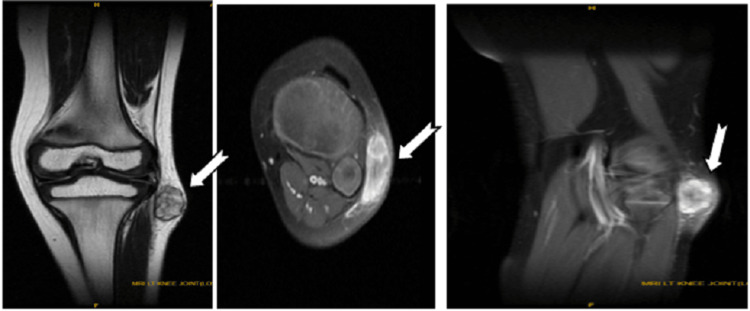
MRI with contrast of knee showing the tumour
